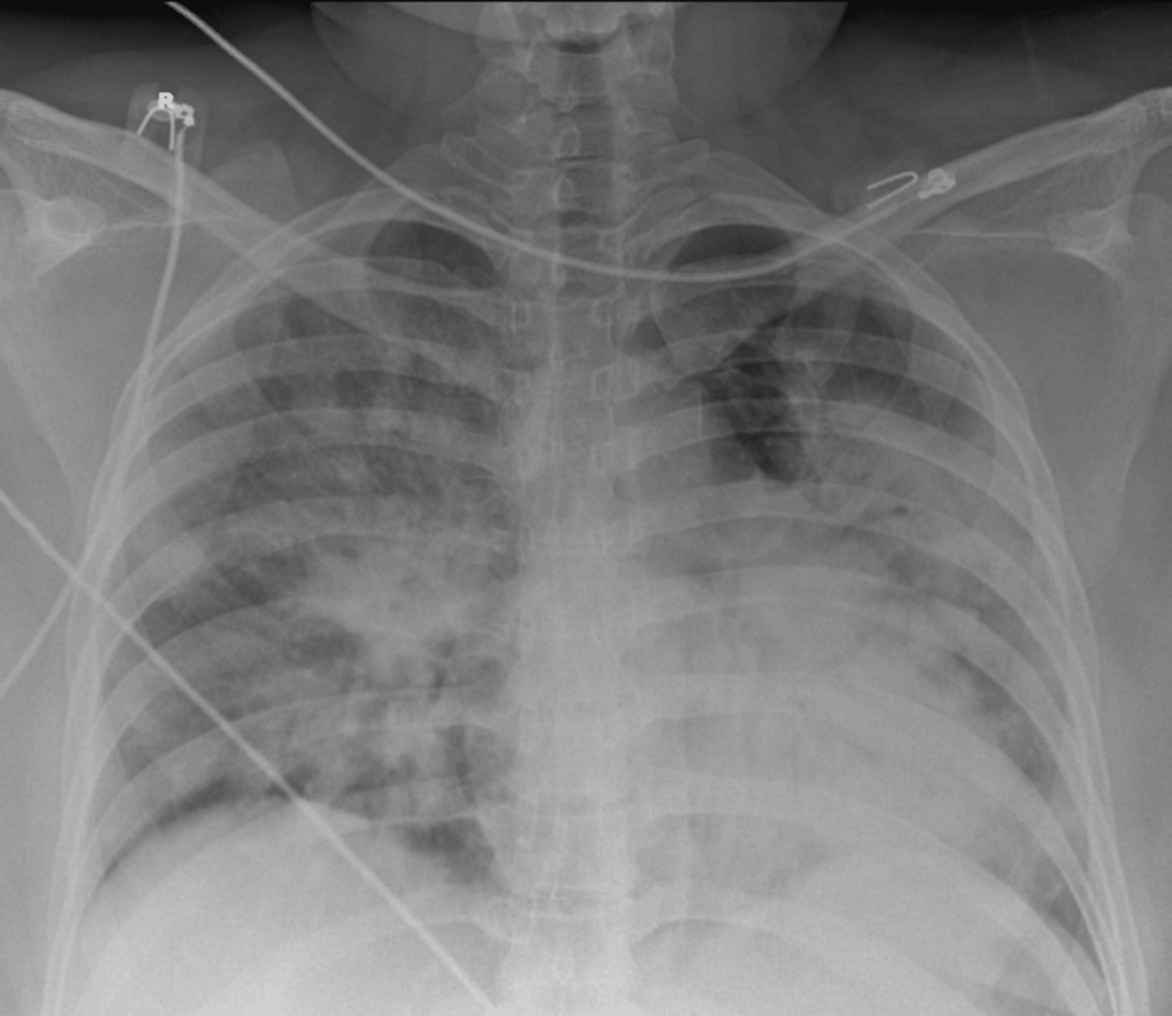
Chest X-ray showing diffuse parenchymal infiltrates consistent with ARDS. ARDS, acute respiratory distress syndrome
